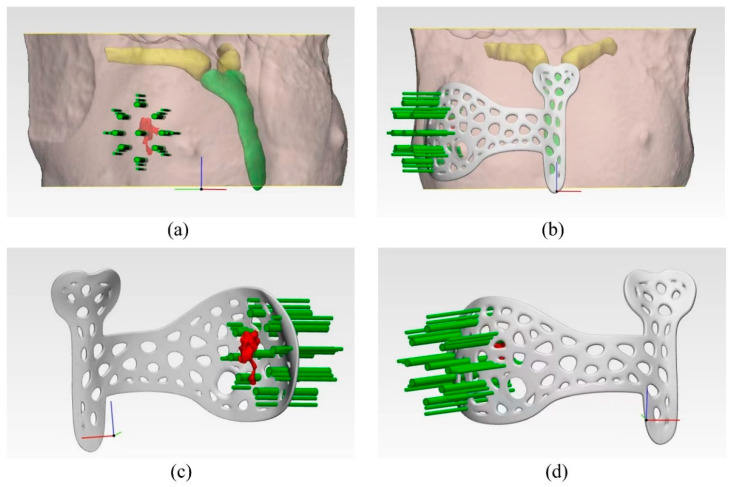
Design of the breast surgery guide based on the 3D segmented model that displays the tumour location and surrounding tissues: (a) Determination of tumour resection boundary. The middle point of the longest axis of the tumour was selected as the centre point of the tumour, based on which a circle indicated by green columns was drawn with a diameter slightly extended by 0.5 cm from the MRI tumour boundary to ensure a safe margin. (b) The shape of the surgery guide was tailored to fit precisely with that of the patient’s breast and surrounding tissues. A circular hole located at the nipple was generated as the main positioning point when the surgeon applied the device onto the surface of the breast. The manubrium was chosen as the second positioning point to promote localisation accuracy. (c) The tumour-side view of the surgery guide containing quantitative information about tumour extent and resection margin. (d) The front view of the surgery guide.
